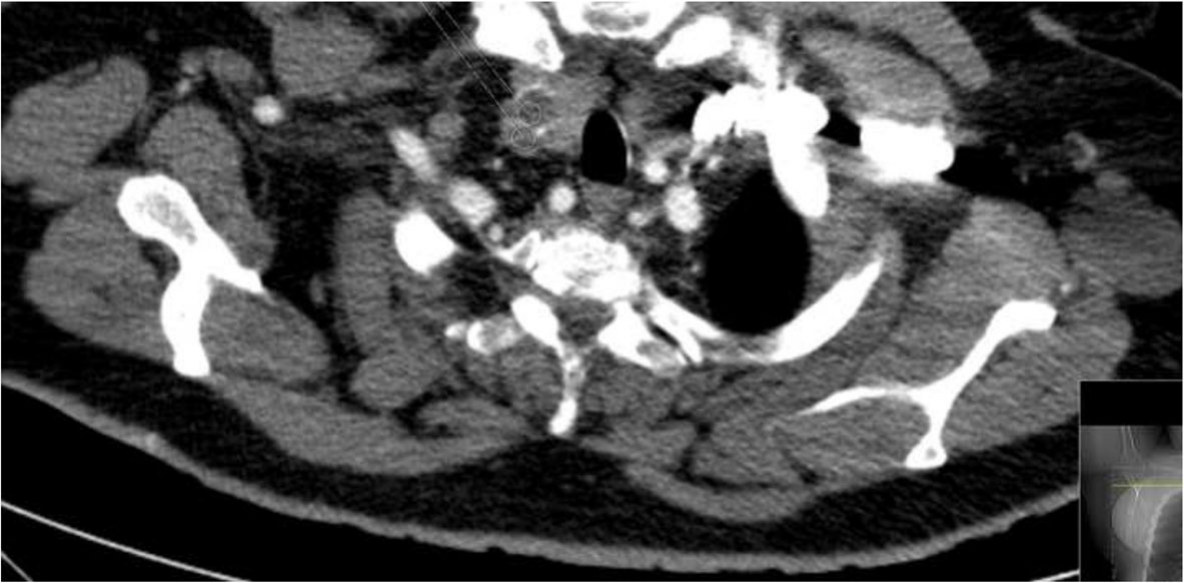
The CT image shows two ROIs in the thyroid nodule. Abbreviations: CT, computed tomography; ROI, region of interest
